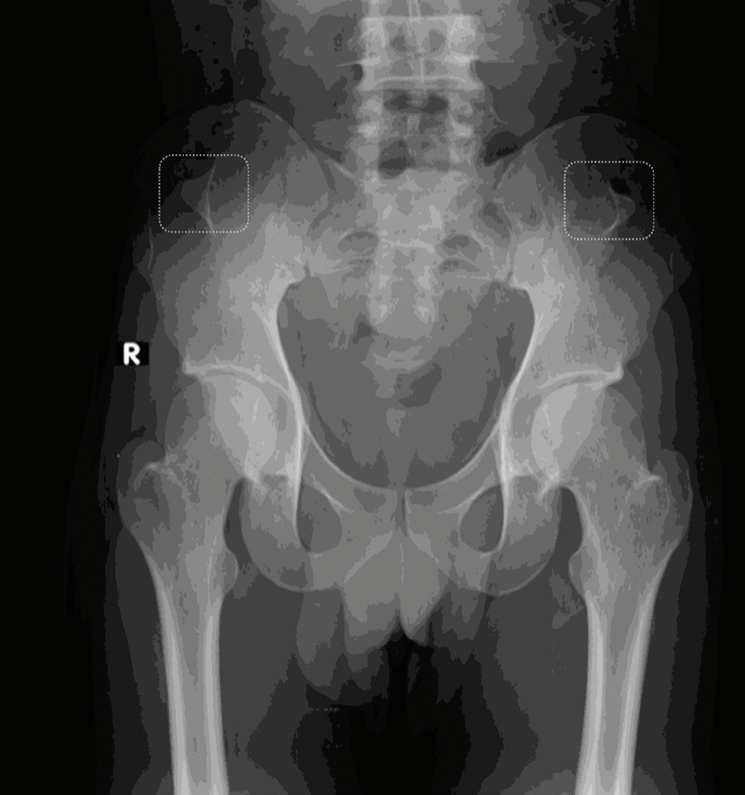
Pelvic X-ray showed bilateral iliac horns.
